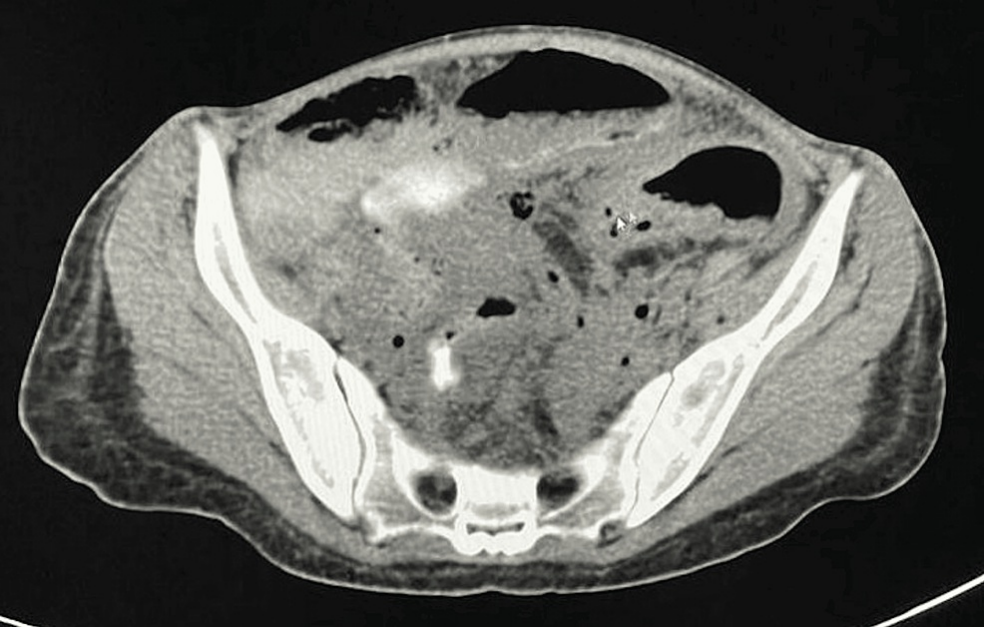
Pre-operative CT scan abdomen and pelvis CT scan with contrast showing pneumoperitoneum, free fluid in the abdomen with septations in the pelvis, and multiple wall-enhancing pockets of collections. Pneumatosis intestinalis is seen in the wall of transverse colon.
